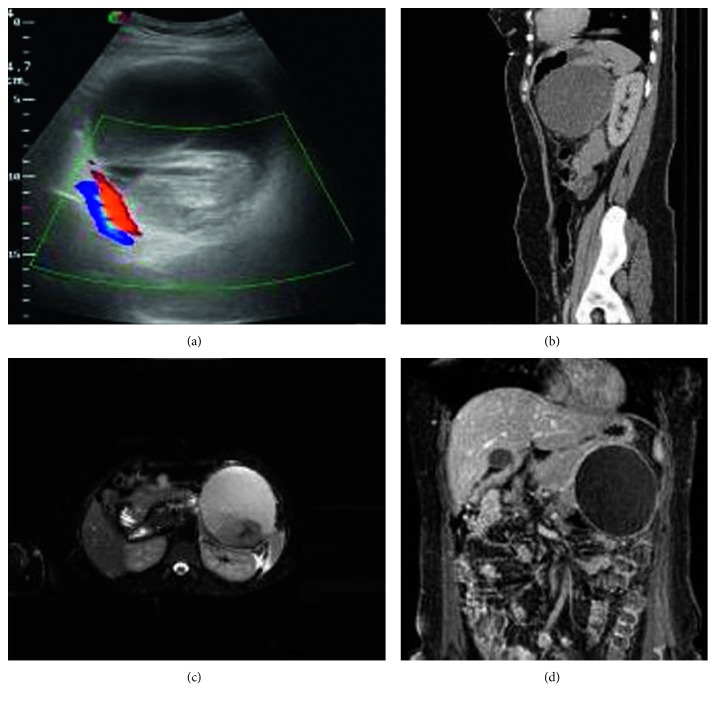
(a–d) Ultrasound imaging examination.
